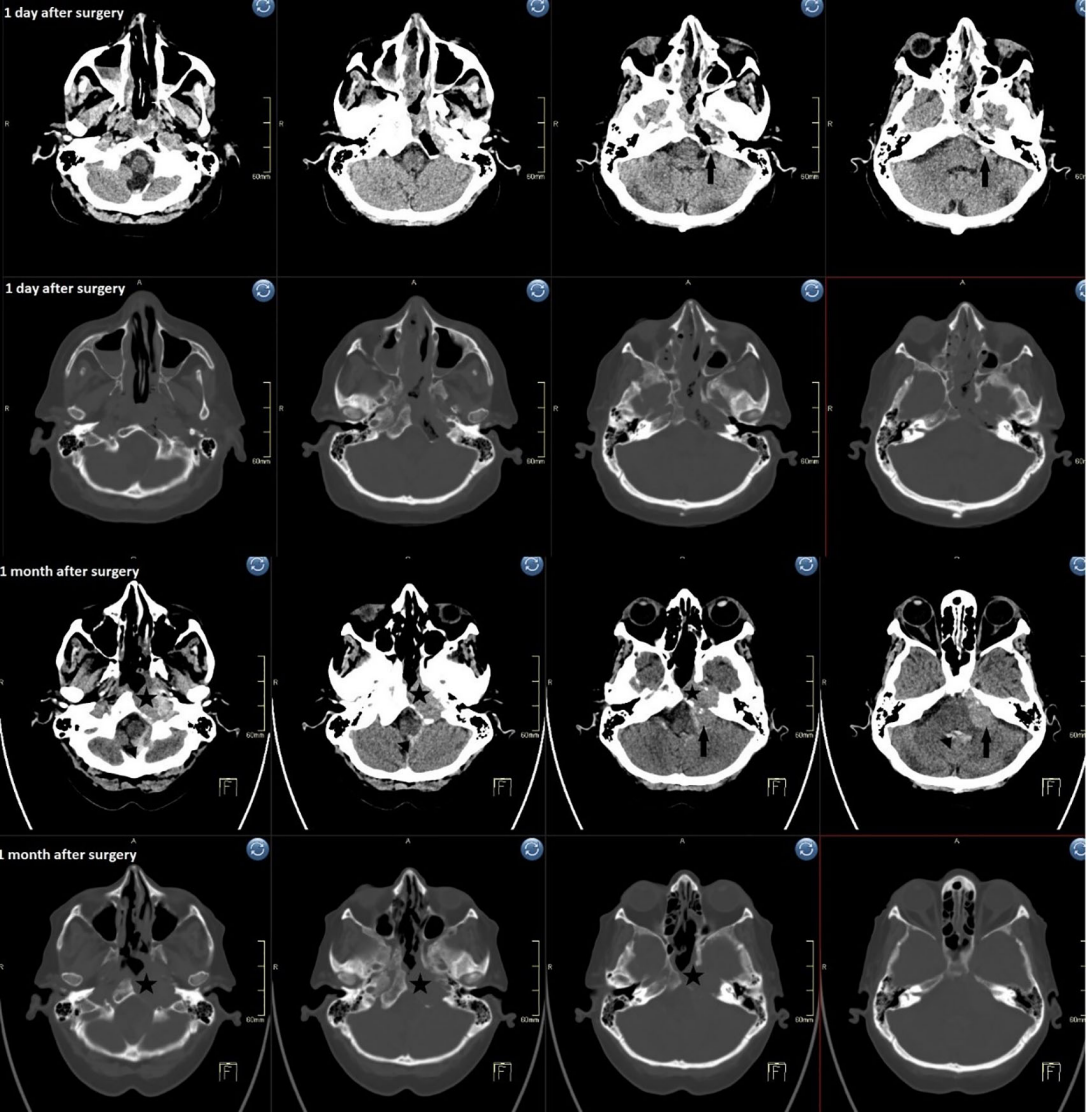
Postoperative CT 1 day (1st and 2nd row) and 1 month (3rd and 4th row) after surgery shows rapid regrowth of the tumor, especially the 7 mm intradural residual. The sudden deterioration started with headache, nausea and vomiting. CT showed SAH in the perimedullary cistern and blood in the fourth ventricle. *(star: tumor regrowth, arrow: 1st row small residual and 3rd row residual enlarged, triangle: haematocephalus in 4th ventricle)*.
